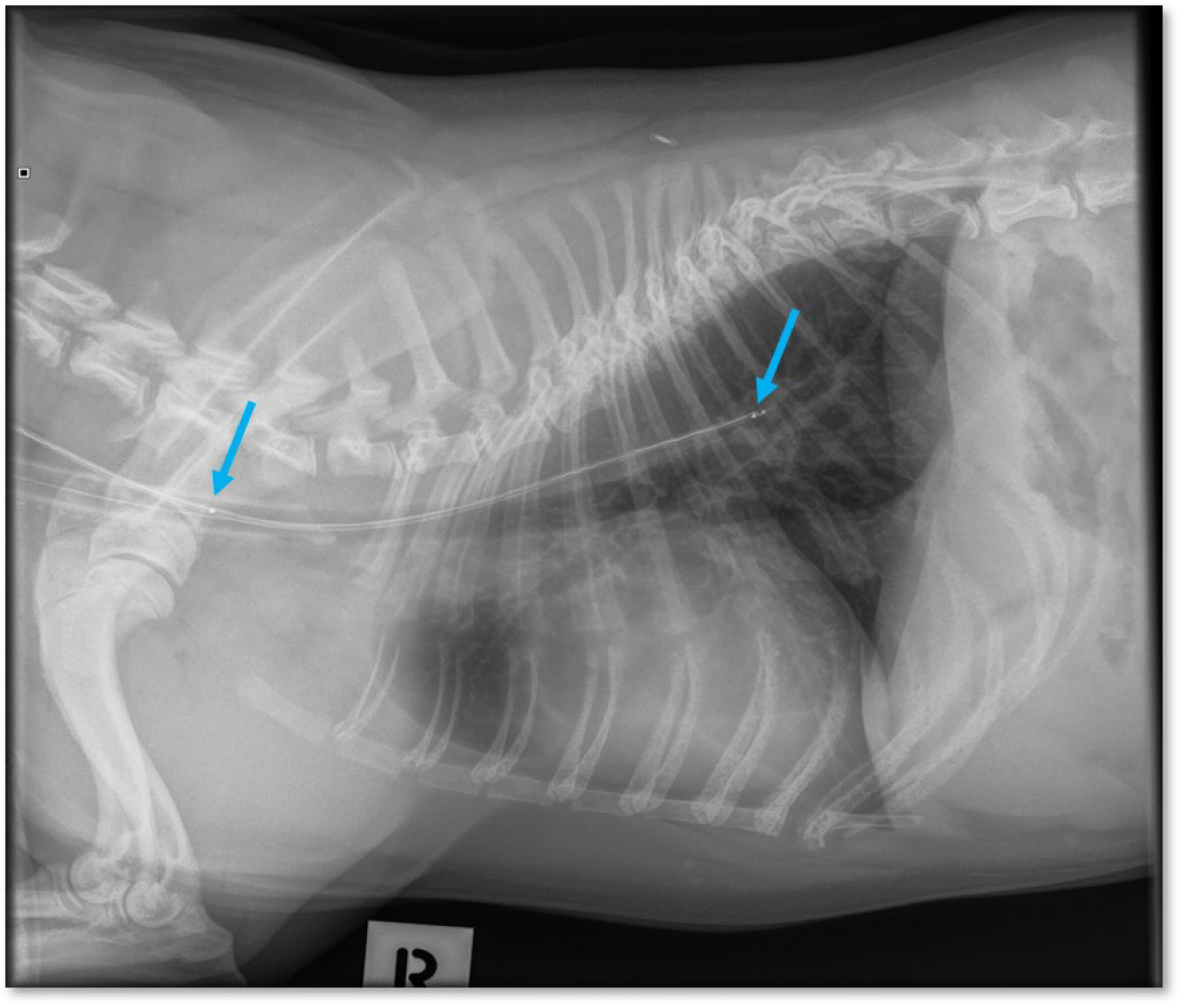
Right lateral thoracic radiograph of a 1‐year‐old French bulldog showing the position of the esophageal probe with the proximal and distal sensor location (arrows)
